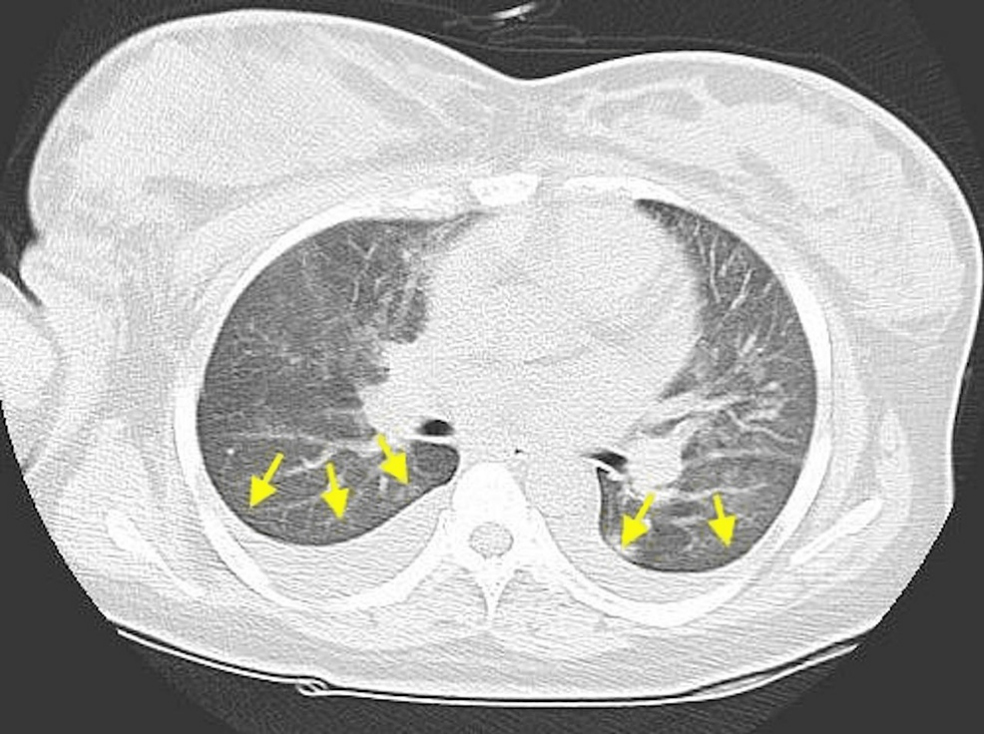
CT image of the chest Bilateral pleural effusions (yellow arrows)
